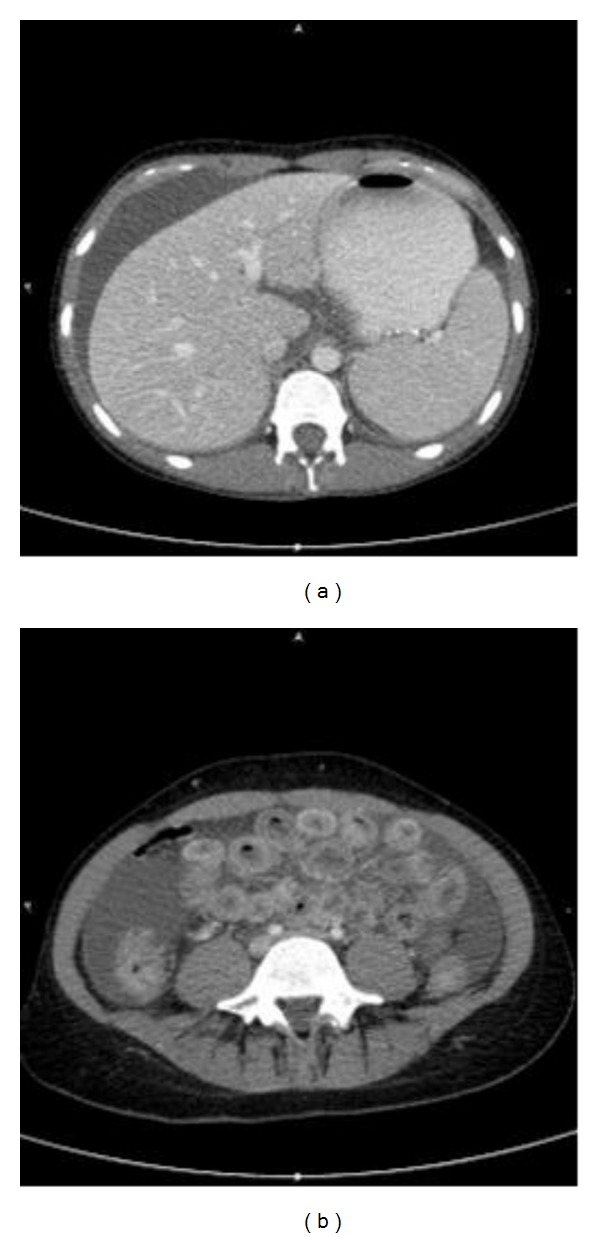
Computed tomography of abdomen and pelvis showing large amount of ascites and thickened small bowel wall. No pelvis or peritoneal mass identified.
